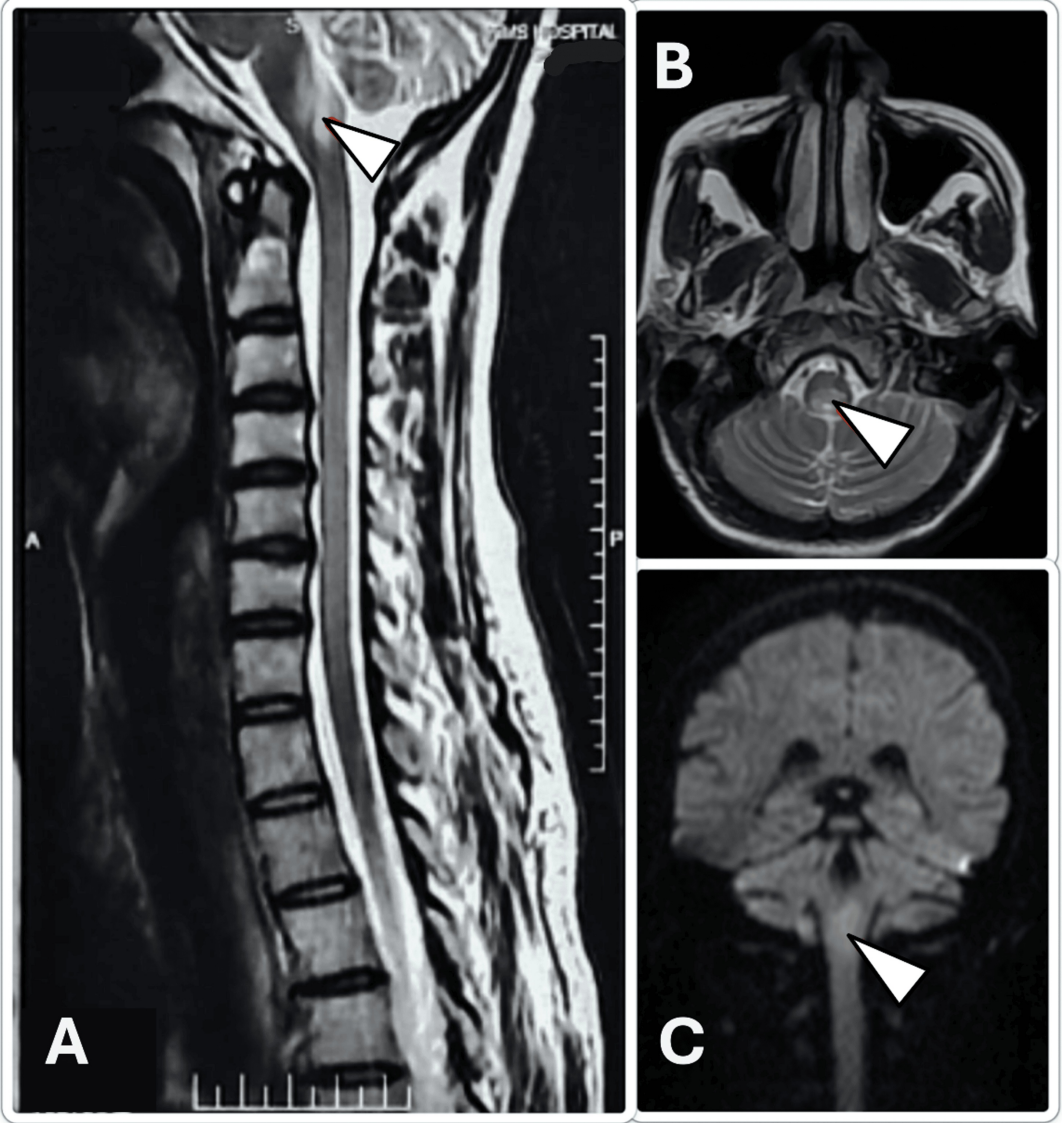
MRI of the brain with a screening of cervical spine showing FLAIR hyperintensities (white arrows) involving various regions of the dorsal medulla, cerebellum, pyramidal decussation, and inferior olivary nucleus. A. Sagittal view. B. Axial view. C. Coronal view. MRI, magnetic resonance imaging; FLAIR, fluid-attenuated inversion recovery
